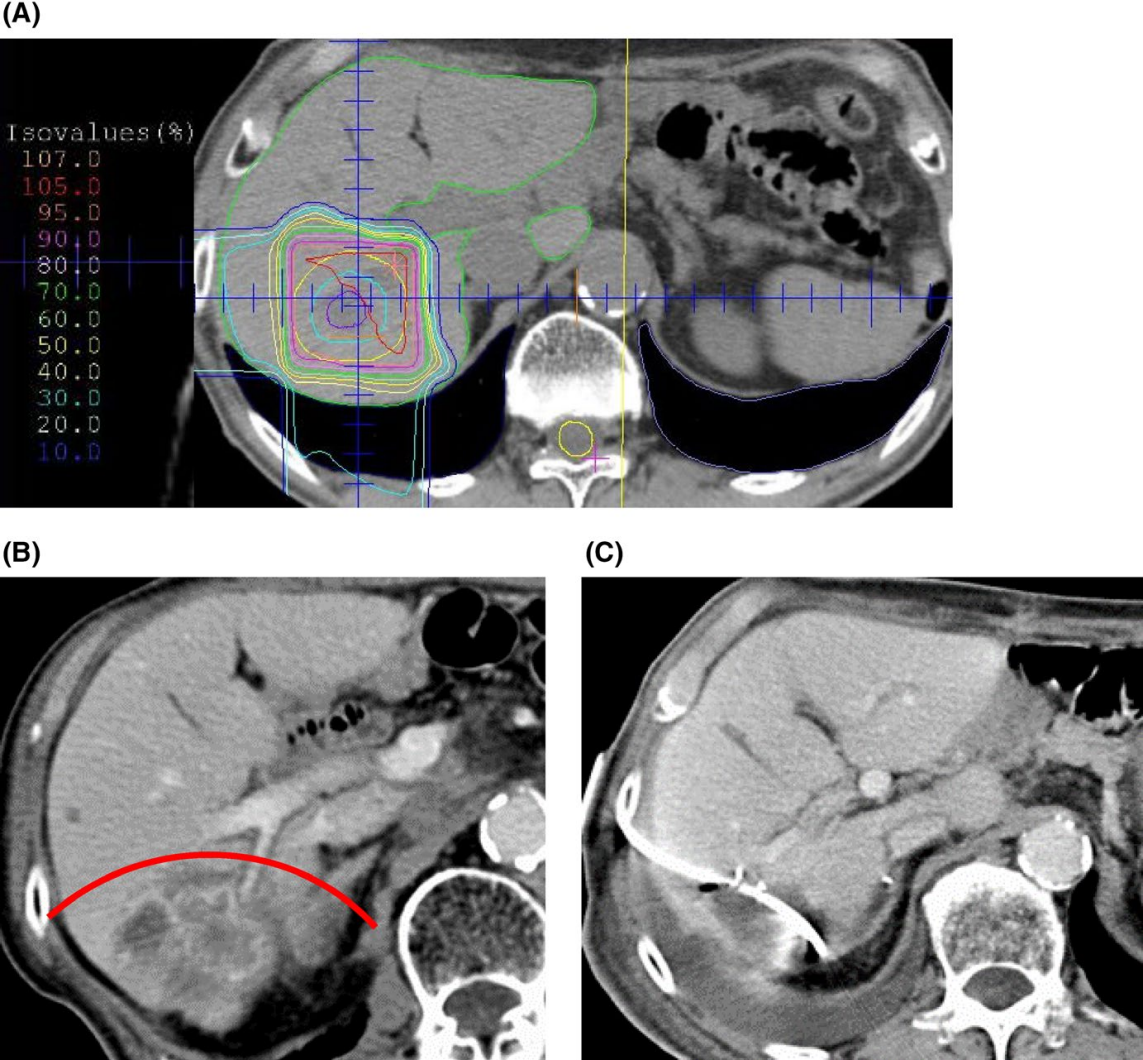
The case of refractory bile leakage (case No. 4). (A) Dose distribution in radiation planning for segment 6 lesion. (B) Computed tomography image at local recurrence. Red line indicates parenchymal resection line performed. (C) Drainage tube placement for postoperative bile leakage
